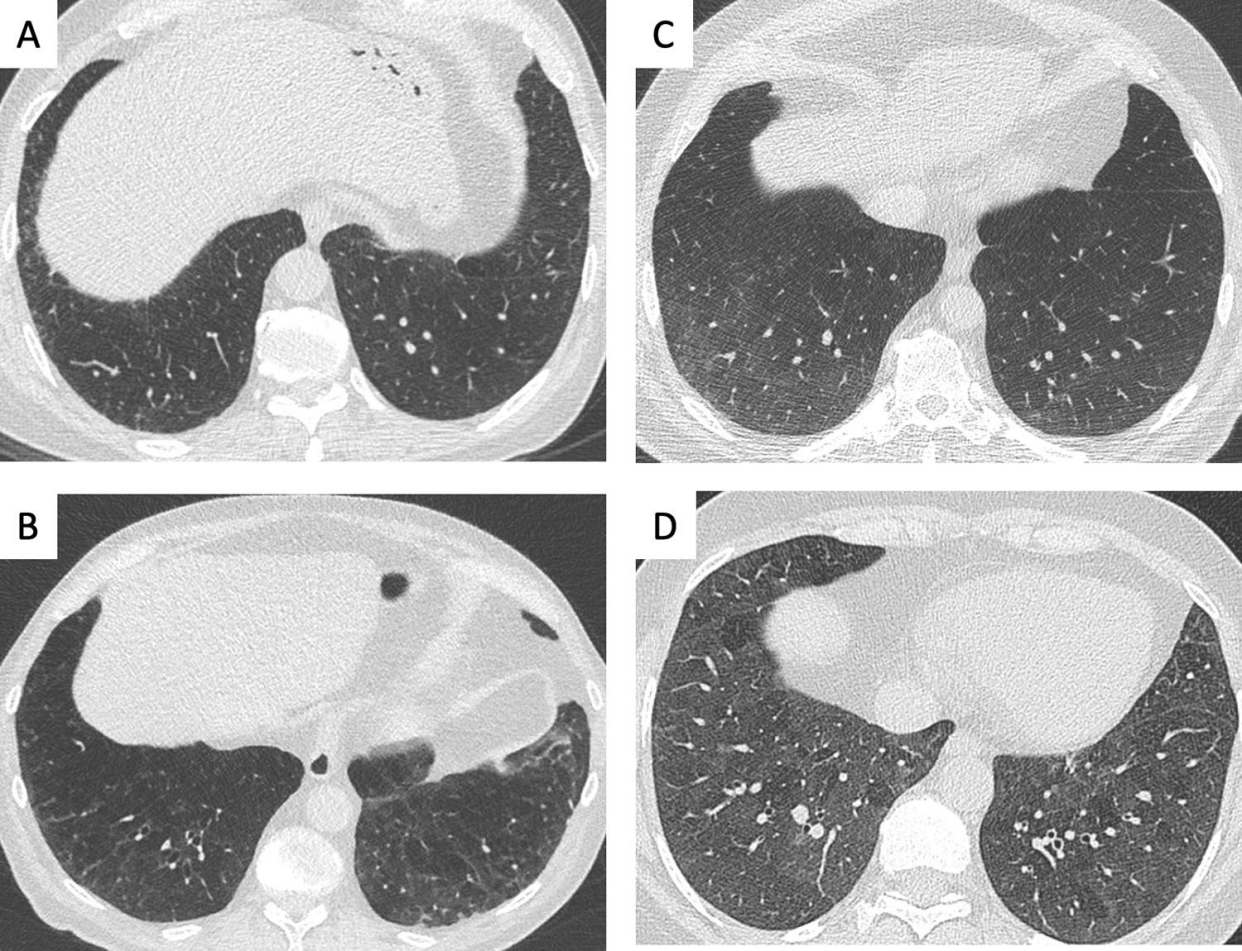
A–D. Collateral (smoking-related) findings in screening LDCT:interstitial lung abnormalities with varying extent and morphology.Axial CT image at the level of mid-lower chest showing different patterns of interstitial lung abnormalities with varying severity: A minor reticulation in right lateral sulcus; B reticulation with signs of bronchiolar traction in the lower lobes; C ground-glass opacity with mild extent in the lower lobes; D ground-glass opacity with extensive distribution in the lower lobes, associated with minimal areas of parenchymal sparing with lobular distribution. These findings variably represent smoking related disease, with either reversible or irreversible behaviour worth of multidisciplinary discussion
